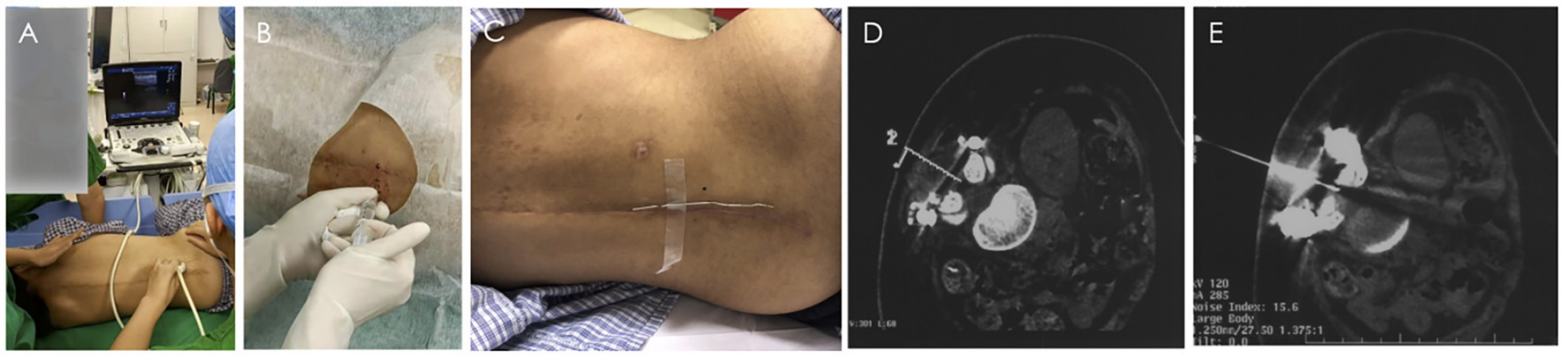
Nusinersen intrathecal injection process under the guidance of B-ultrasound and CT. (A,B) Real-time ultrasound-guided lumbar puncture. (A) Ultrasound probe position of paramedian sagittal oblique scan at the level of lamina. (B) Local anesthesia before intrathecal injection. C-E CT-guided lumbar puncture. (C) Place a metal positioning wire at the posterior midline around the lumbar spine. (D) Determine the needle insertion route through CT scan, and calculate the needle insertion point on the body surface and needle insertion angle. (E) The puncture needle successfully entered the subarachnoid space).
